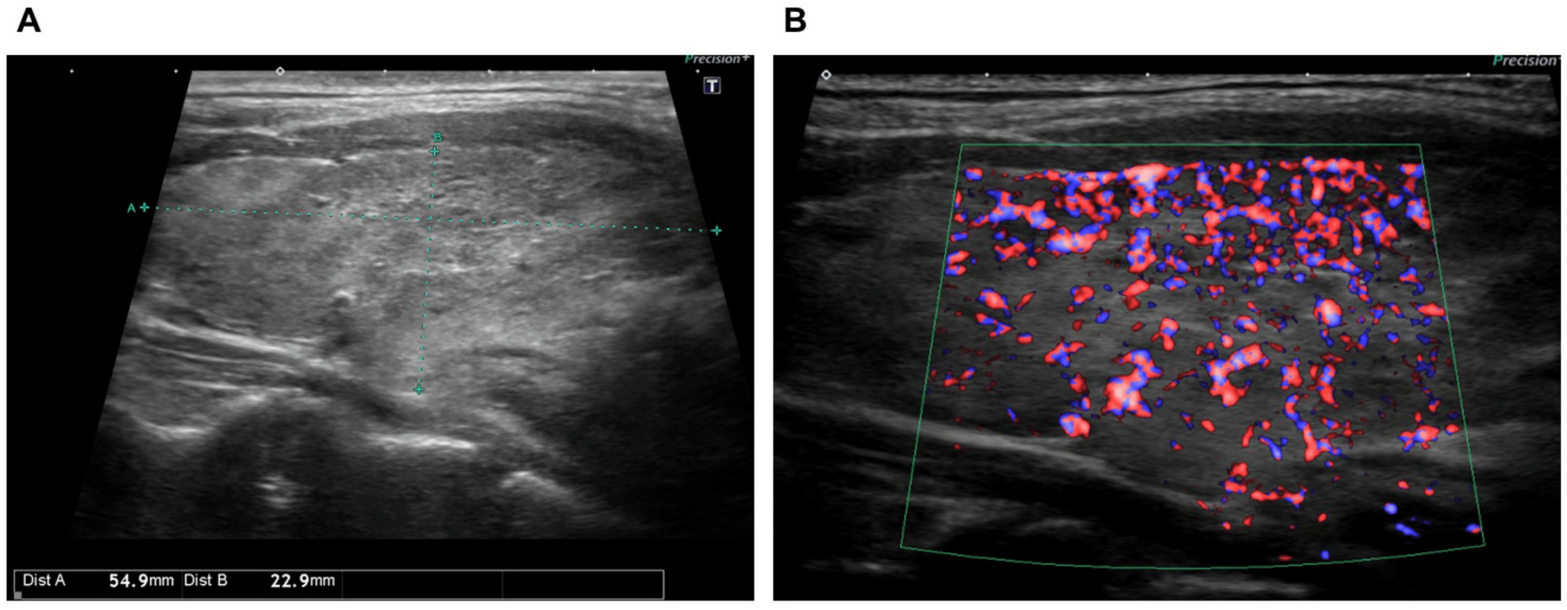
Thyroid ultrasound findings. (A) Thyroid ultrasound showing diffuse thyroid enlargement.
